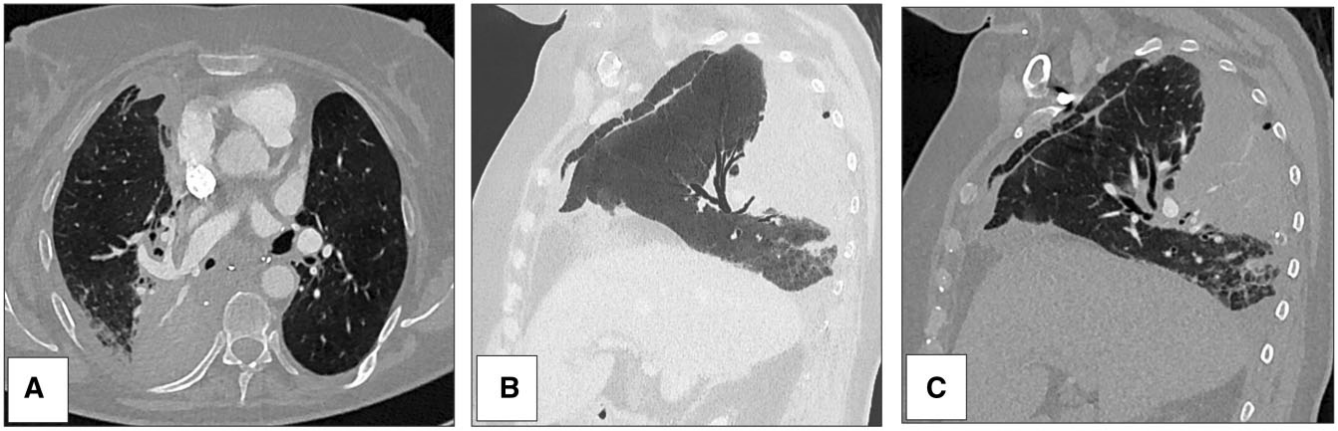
Case no 2: chest CT scan at day 6 with axial view (A) and sagittal views (B and C). A middle and lower lobe malposition with a 180° lobar tilt were revealed. Middle lobe positioned in the back part of pleural cavity presented signs of infarction. Diaphragmatic face of right lower lobe was close to back part of the anterior chest wall and Fowler’s segment was in extreme lower part of the pleural cavity. The diameter of pulmonary artery for right lower lobe was also reduced (A).
